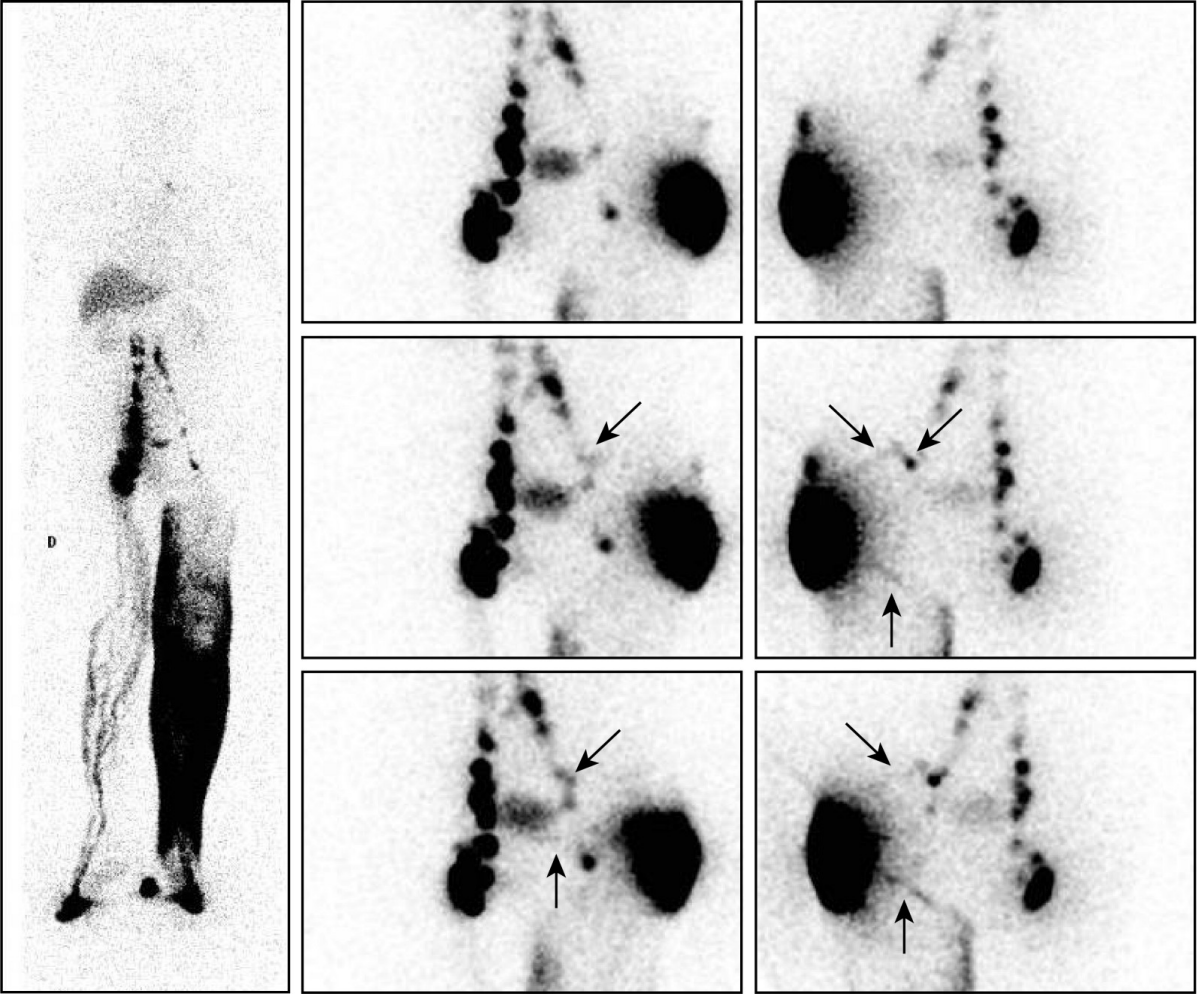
“Intra-abdominal LNs and collateral vessels are shown in a case of secondary LLLE!”. Phase 3 lymphoscintigraphy [left: Anterior WBS obtained after 1 hour of walking [phase 3] at the end of our exam] revealed in a 59-year-old man with left-sided LLLE secondary to surgery and radiotherapy for liposarcoma of the thigh the following:
